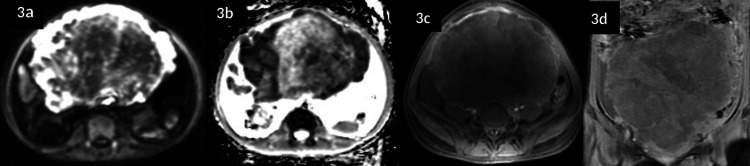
Preoperative diffusion-weighted and post-contrast MRI a and b: Marked peripheral restriction, reflecting high cellularity, with low apparent diffusion coefficient (ADC) values; c and d: Postcontrast sequences that show a heterogeneous mild enhancement with large centro-tumoural necrosis
